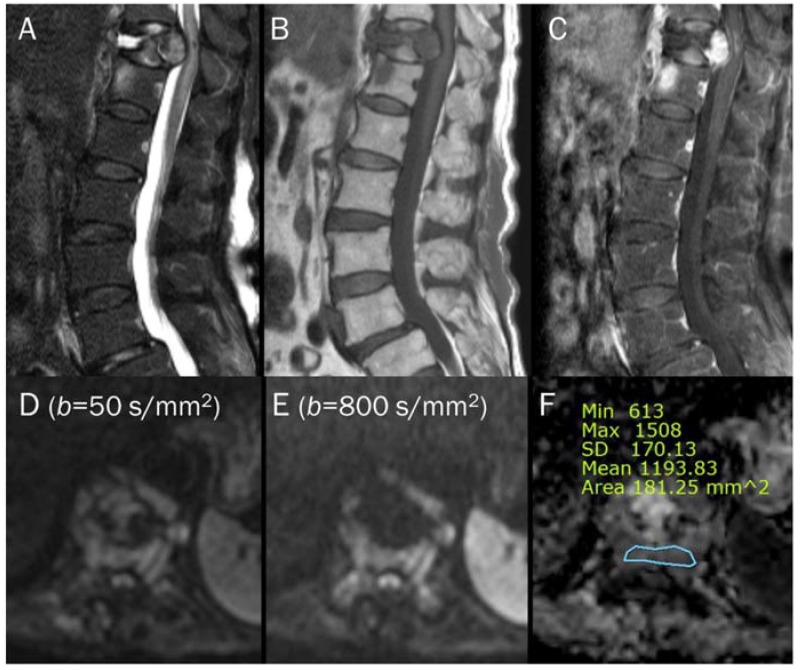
An 87-year-old female identified as pathologic compression fracture with renal cell carcinoma at 11th thoracic vertebra. Conventional sagittal MRIs (A: T2-weighted fat-suppressed, B: T1-weighted, C: T1-weighted enhanced) show severe vertebral collapse with posterior bulging contour, as well as frame-like enhancement around the fluid cleft. (D,E) DWI with *b*-values of 50 and 800 s/mm^2^ exhibit progressive signal increase in the enhancing cellular portion with (F) a reduced signal in the ADC map with mean ADC value of 1193.83 μm^2^/s, suggesting “restricted” diffusivity and interpreting it as bone metastasis. Note that the largest ROI is selected to encompass most of the lowest signal intensity on the ADC map.
